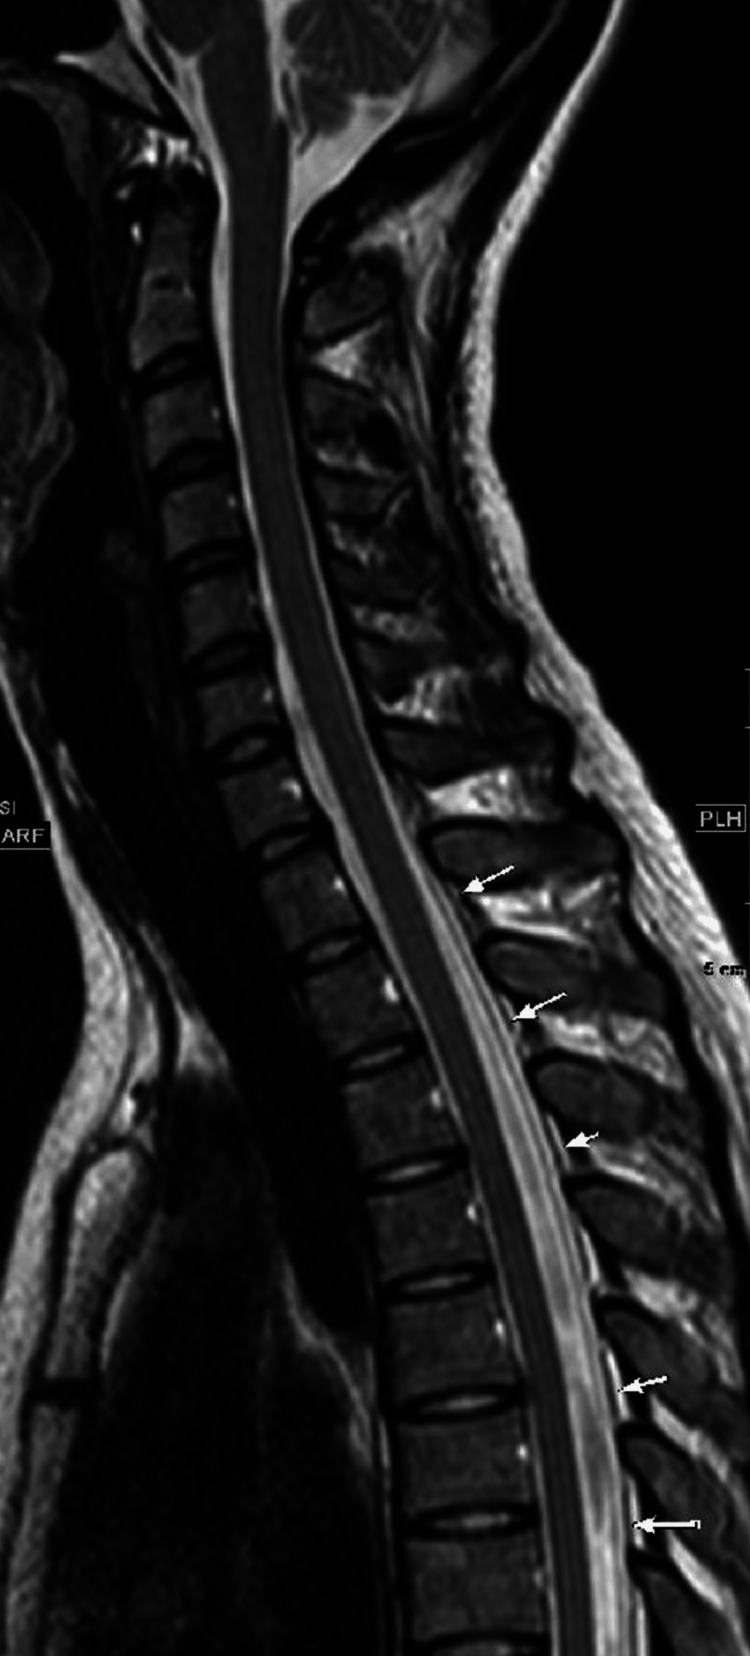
Magnetic resonance imaging myelography sagittal T2 shows fluid accumulation in the epidural space around T1–7
